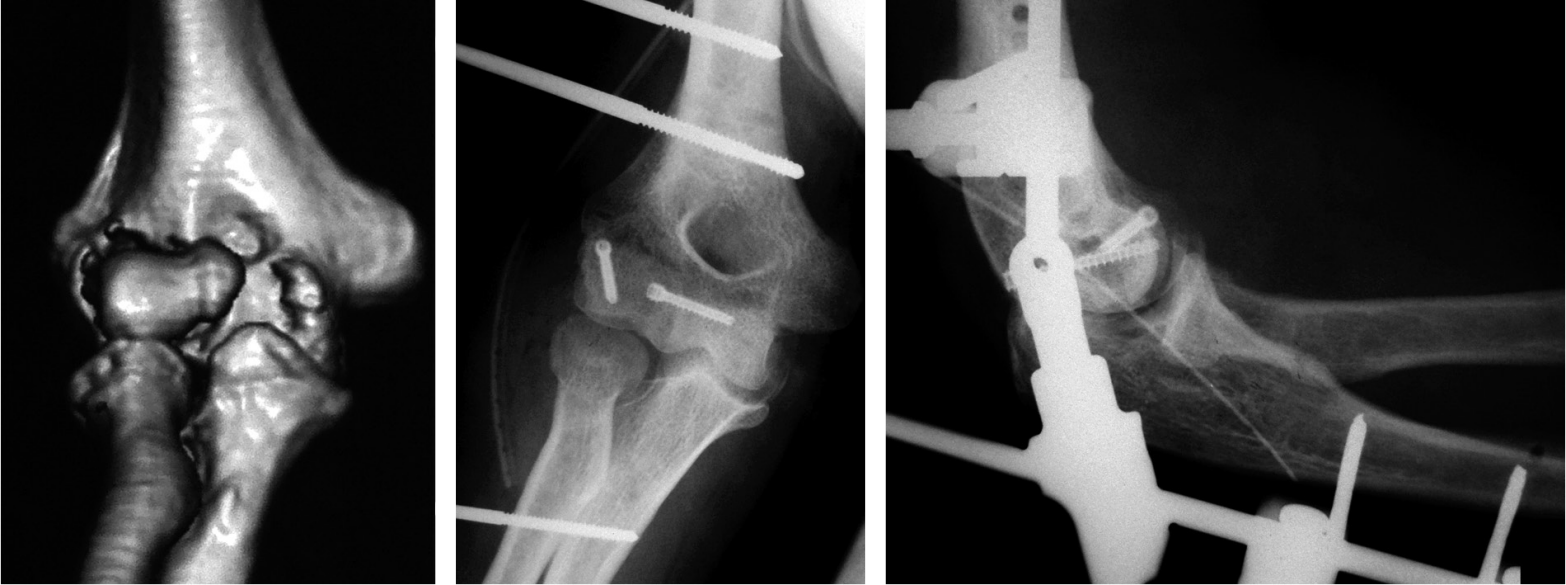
Case 1. 3-D CT reconstruction showing displaced fracture of the capitulum humeri and trochlea. Internal fixation screws supplemented with a hinged external fixator.
